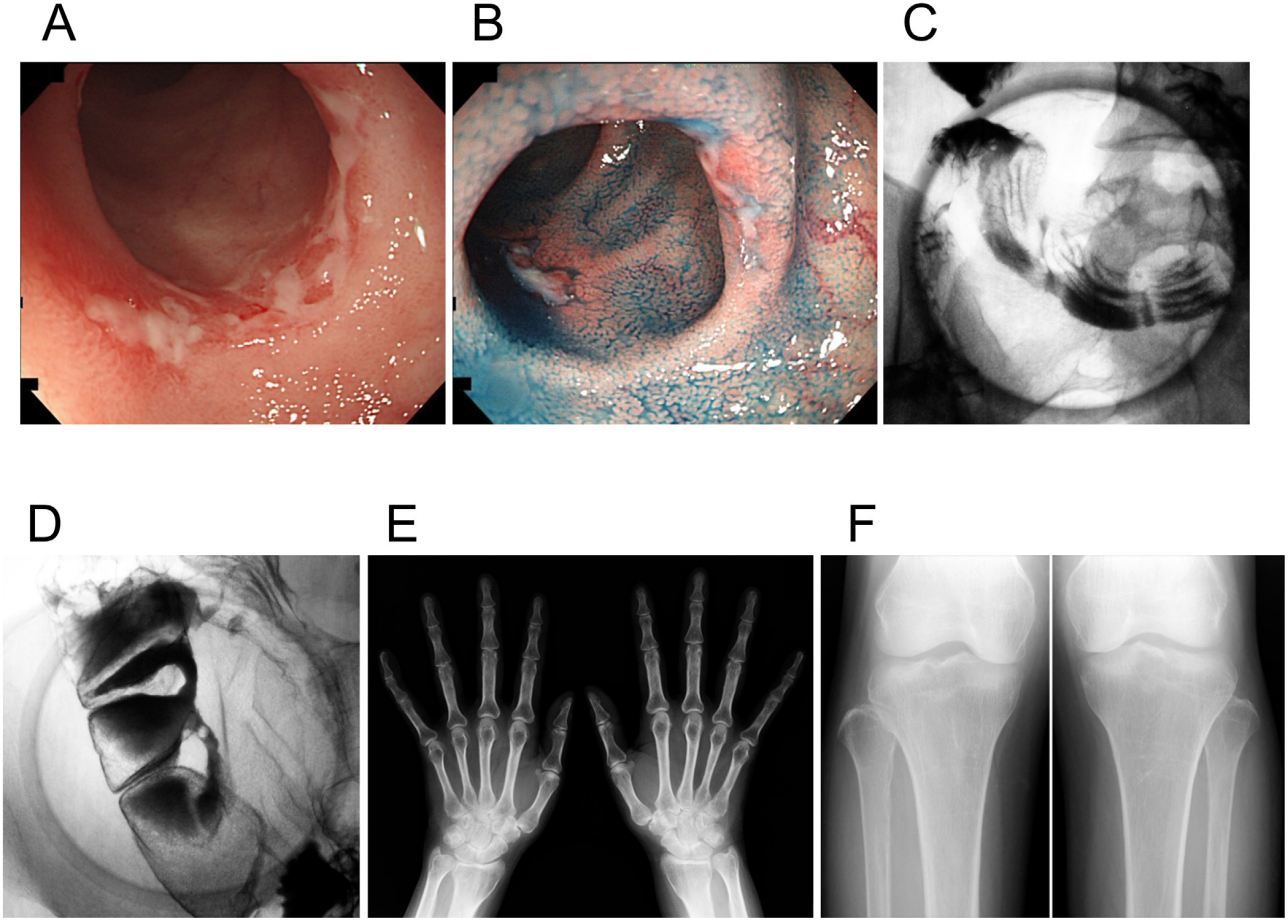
Clinical images of an individual with chronic nonspecific multiple ulcers of the small intestine (patient A-V–2). (A, B) Retrograde ileoscopy shows active circular and oblique multiple ulcers with mucous exudates in the ileum. (C, D) A barium follow-through examination with compression shows multiple circular barium flecks (C), eccentric deformities, and strictures (D) in the ileum. (E, F) Radiographs of the hands and tibiofibulae show no obvious abnormalities such as cortical thickening of the metacarpals and periosteal hyperostosis.
